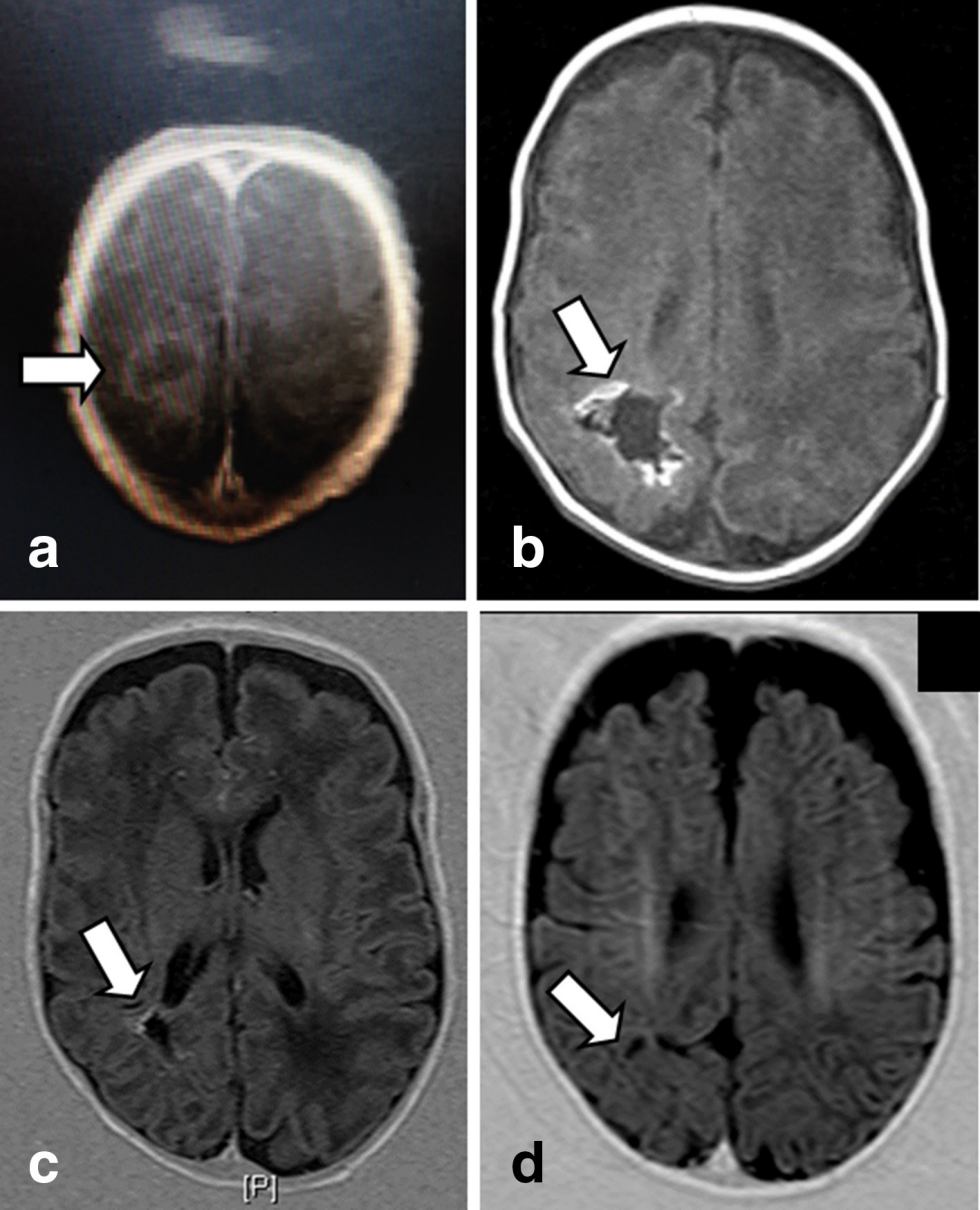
MRI of the infant’s brain abscess. Area of suspected brain abscess indicated by white arrows; (a) day 18 following admission to NICU; (b) 3 weeks after therapy; (c) 6 weeks after therapy; (d) 5 months after therapy.
